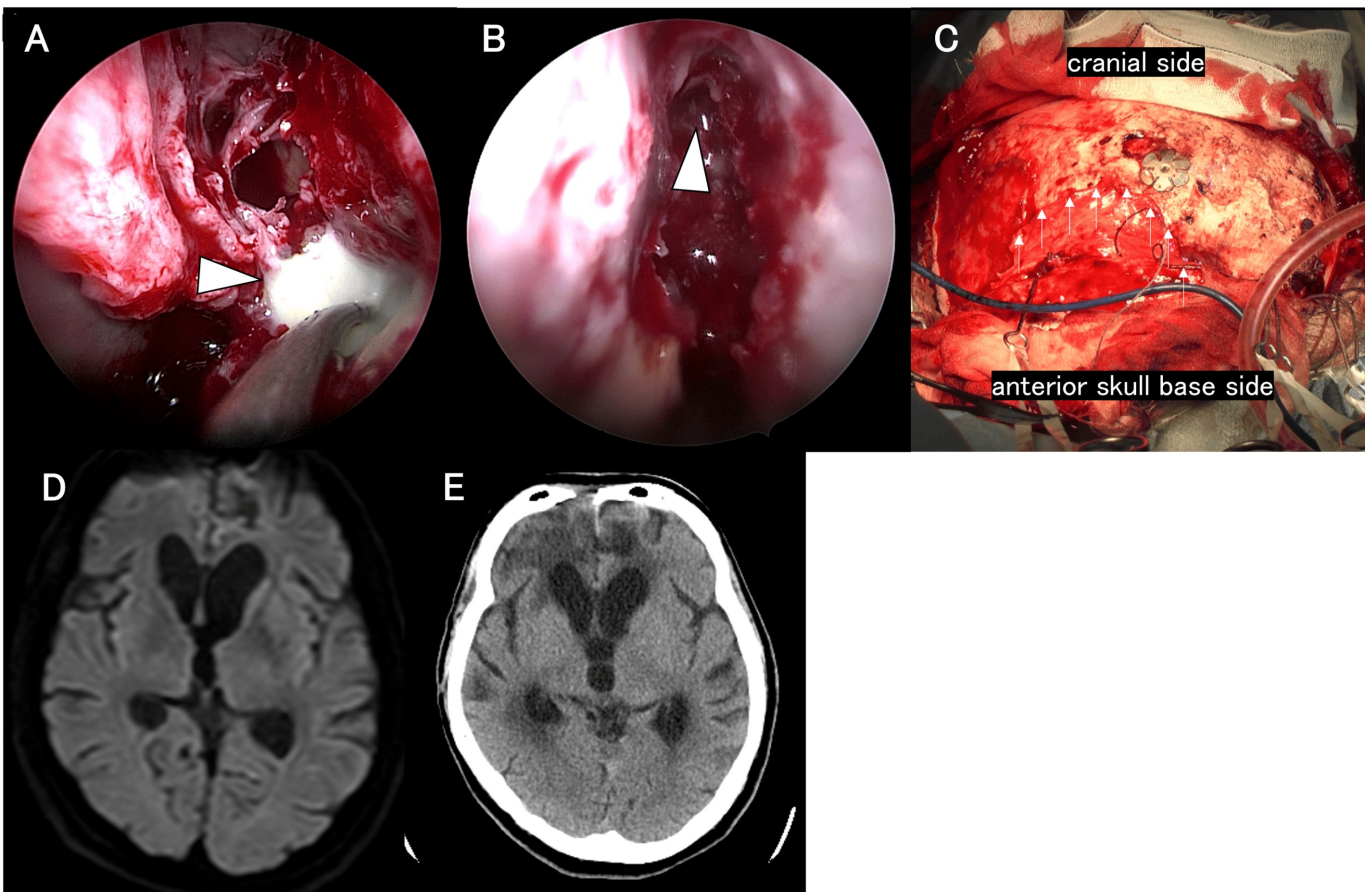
Intraoperative findings (A-C) and postoperative imaging (D, E) following endoscopic sinus surgery and CSF leak repair in Case 2 A: The abscess was observed in the left maxillary sinus (arrowhead). B: CSF leakage from the frontal sinus is confirmed (arrowhead). C: The frontal sinus opening is closed using the periosteum and fascia (arrows). D: Brain MRI-DWI on day 59 reveals no recurrence of the brain abscess. E: Head CT on day 84. CSF, cerebrospinal fluid; CT, computed tomography; MRI, magnetic resonance imaging; DWI, diffusion-weighted imaging
